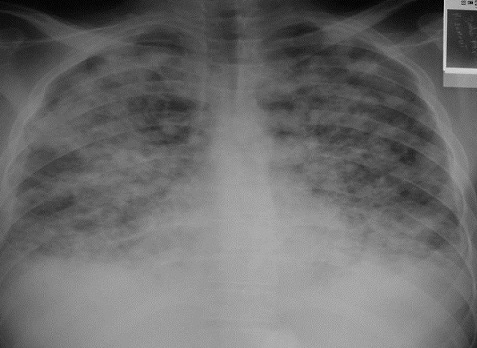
Radiographie du thorax du patient: opacités macro-nodulaires diffuses au niveau des deux champs pulmonaires
